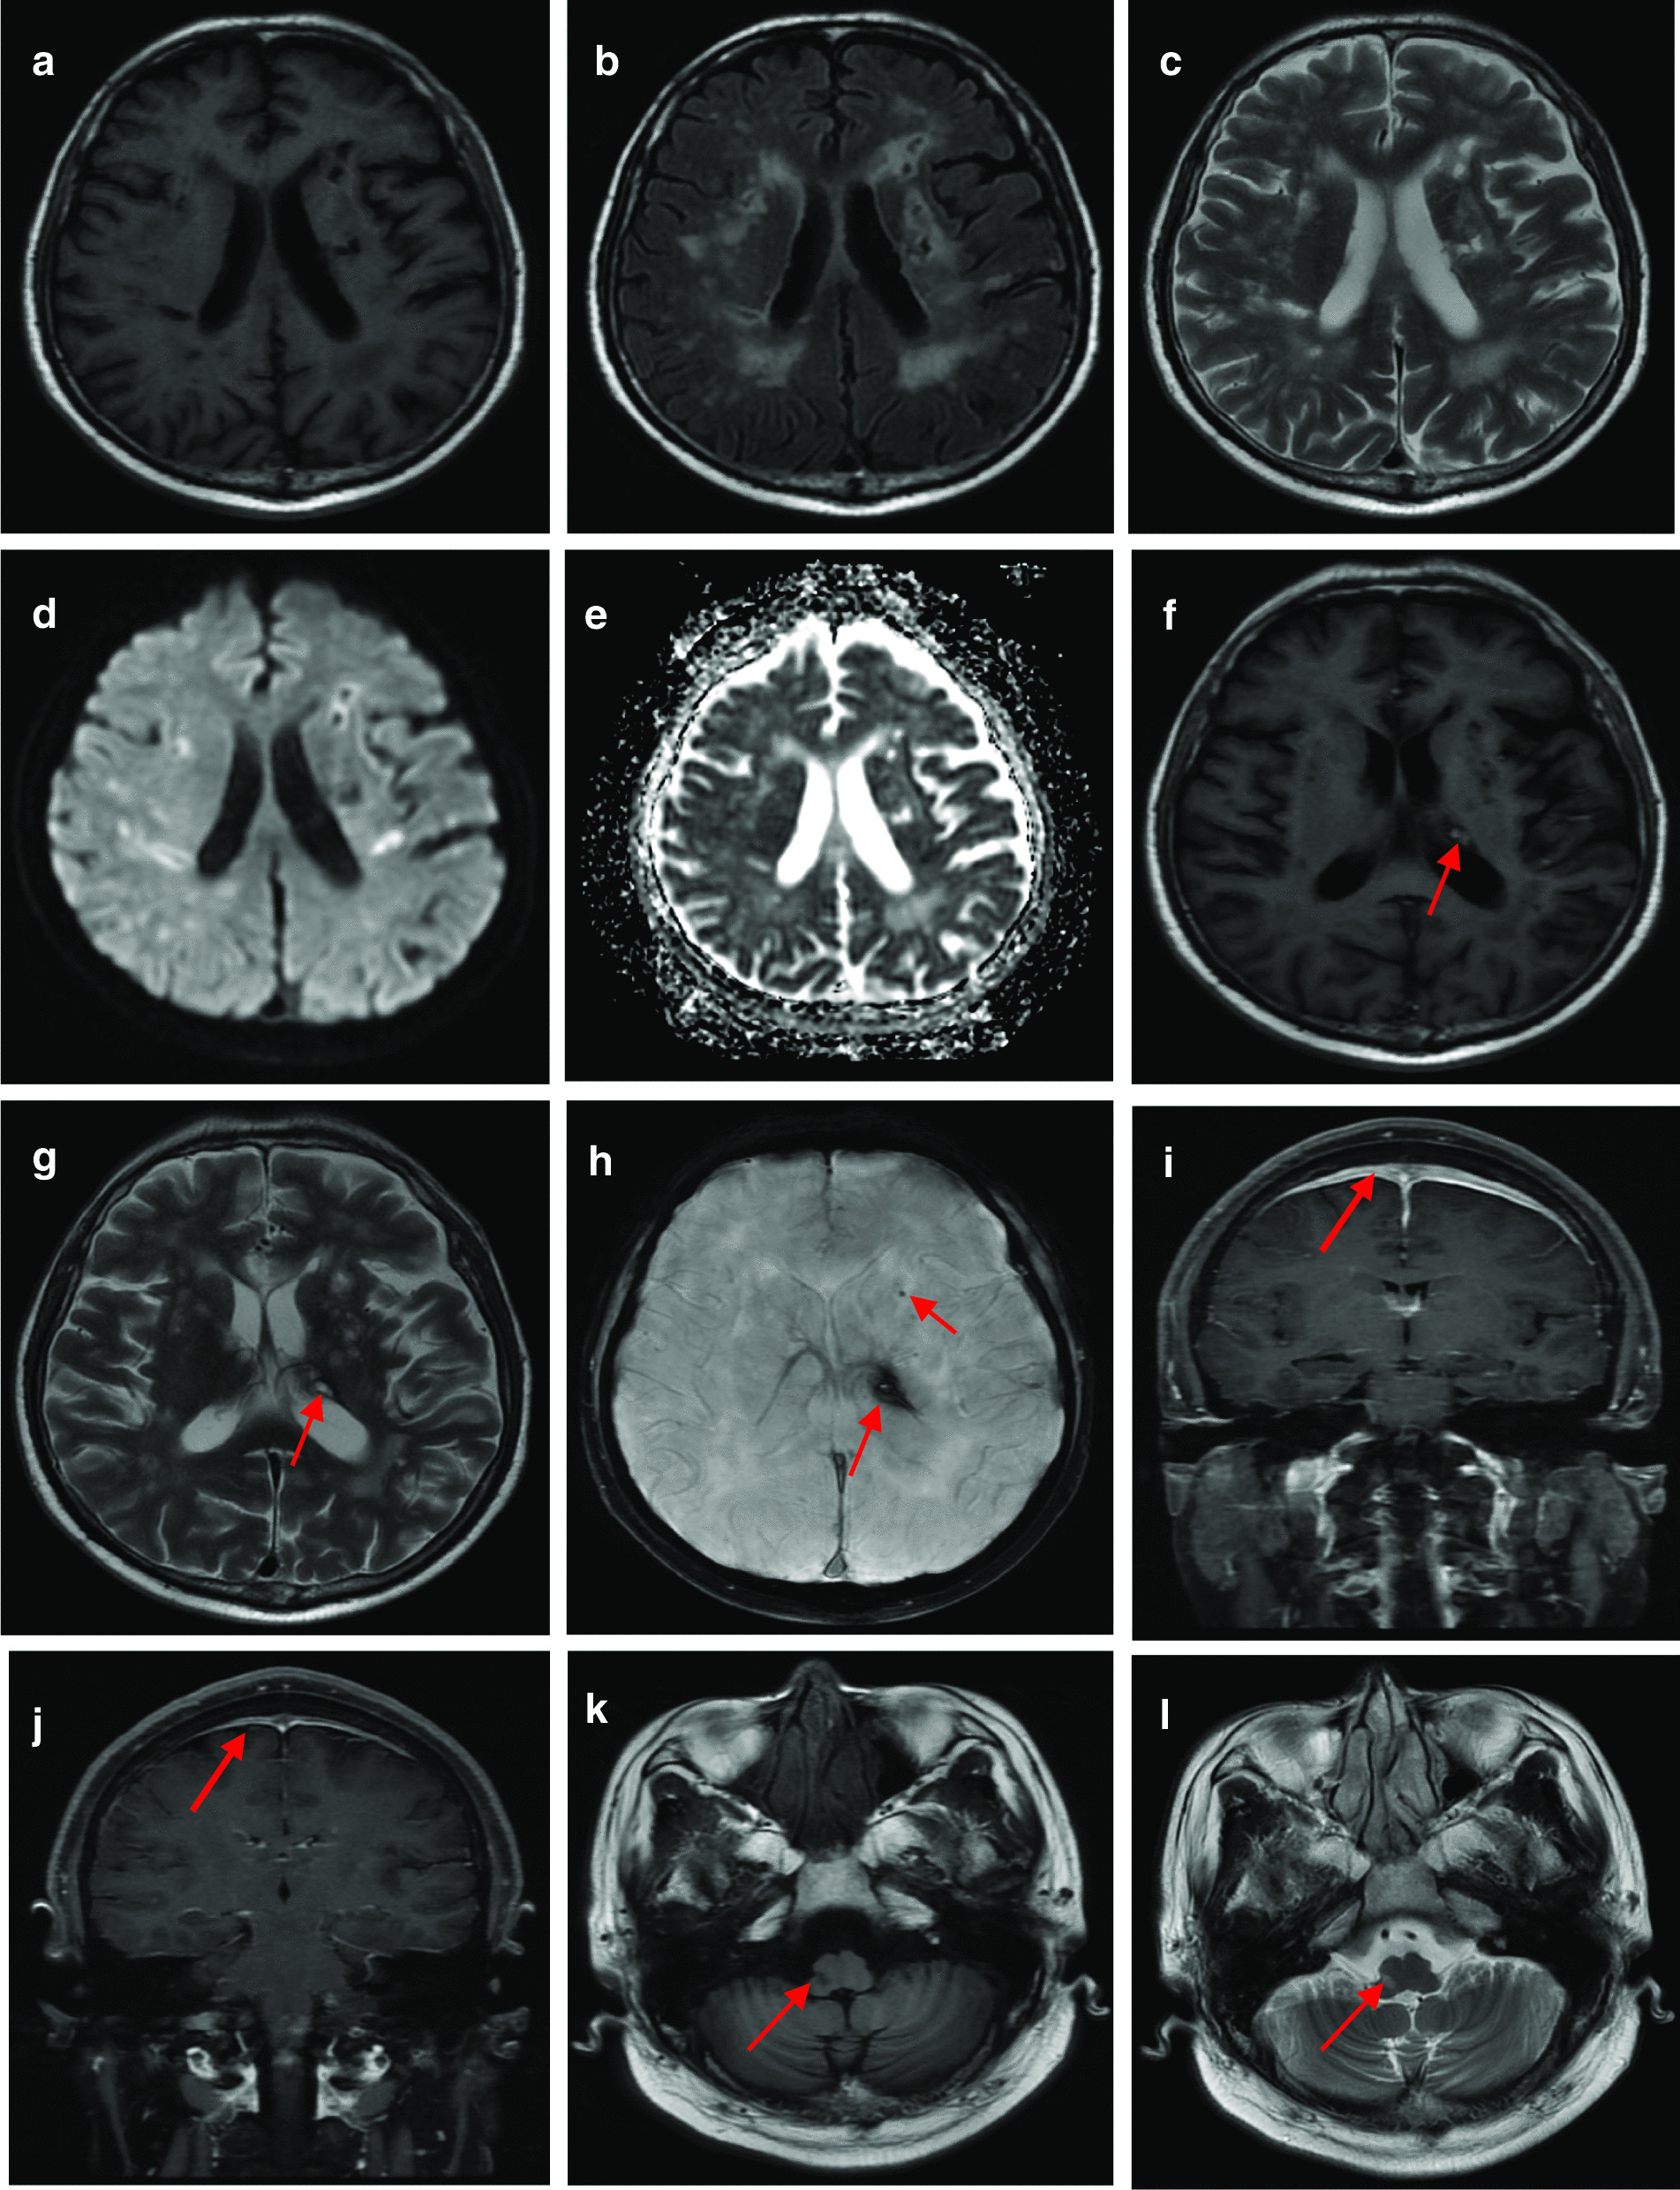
Brain magnetic resonance imaging of EGPA patients with central nervous system involvement. T1-weighted (a), T2 fluid-attenuated inversion recovery (Flair; b), T2 propeller (c), Diffusion-Weighted Images (d), and Apparent Diffusion Coefficient (e) show white matter lesions and multiple cerebral infarctions of Case 19; T1 Flair (f), T2 propeller (g) and T2*-weighted gradient-recalled echo (h) show intracerebral hemorrhages of Case 19. Another patient (Case 11) with hypertrophic cranial pachymeningitis (i) got alleviation of dural involvement after therapy (j). The images of T1 Flair (k) and T2 propeller (l) indicate the medulla oblongata involvement of one patient (Case 18). The corresponding lesions are indicated by the arrows.
